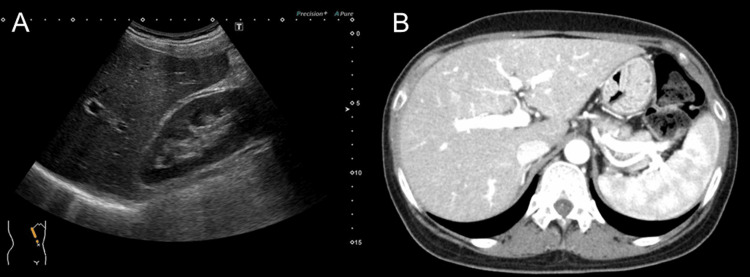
Findings from imaging studies (A) Ultrasonography. (B) Contrast-enhanced computed tomography. They showed no abnormalities in the liver morphology or hepatorenal echo contrast.
